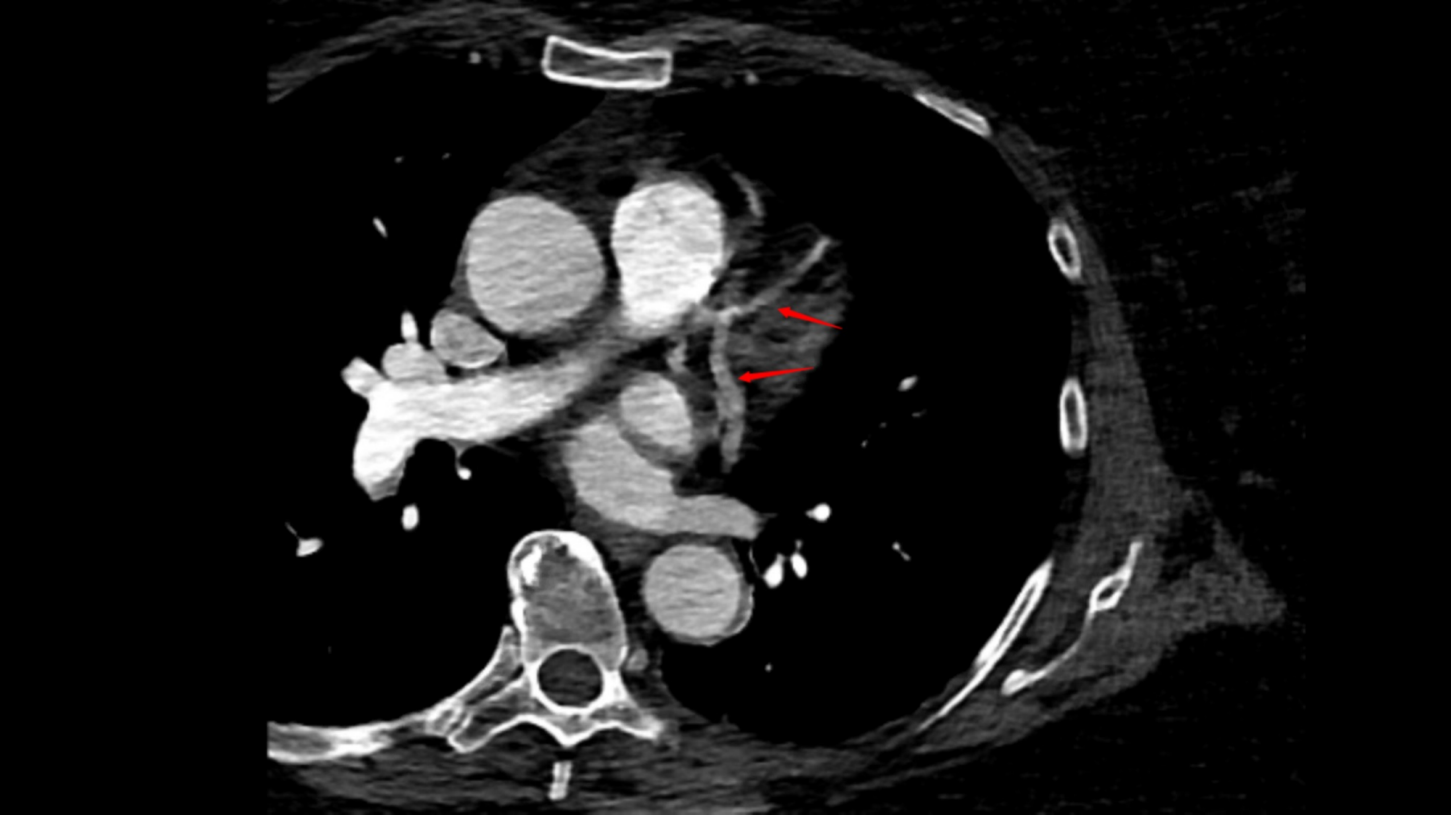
CT scan - view 2 Arrows in the image indicate the LAD artery and RCA. The point of origin of RCA from the LAD artery can be clearly seen CT: computed tomography; RCA: right coronary artery; LAD: left anterior descending
